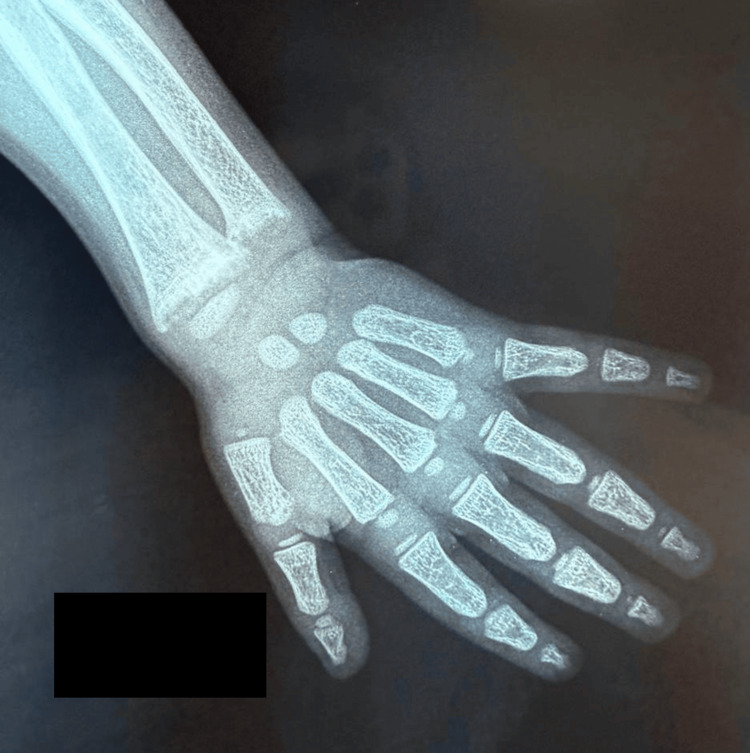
Hand and left wrist radiograph for bone age assessment. Ossification centers are compatible with chronological age. No significant bone maturation delay. Metaphyseal changes consistent with active rickets are noted.
